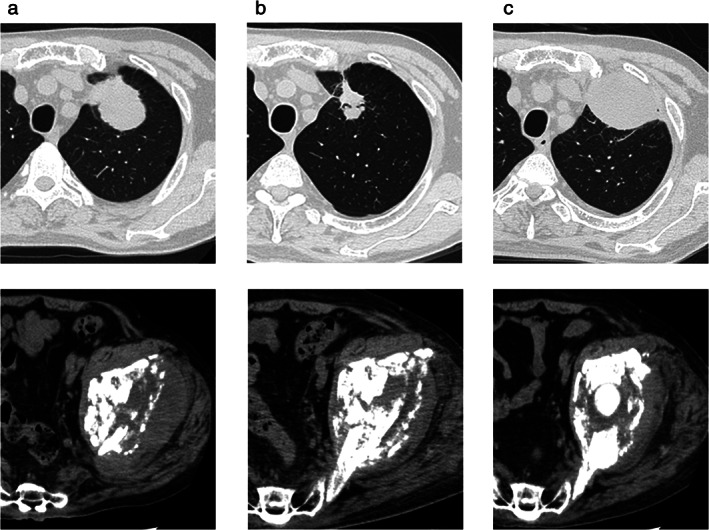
Chest and pelvis computed tomography (CT) scans in a patient with pulmonary sarcomatoid carcinoma. (a) Before treatment with pembrolizumab/carboplatin/pemetrexed, the size of the lung tumor was 50 mm in diameter. (b) After four courses of pembrolizumab/carboplatin/pemetrexed, the diameter of the lung tumor decreased to 26.4 mm. (c) A total of 84 days after initiation of treatment with prednisolone, the diameter of the lung tumor had increased to 62.3 mm. (upper, primary lung tumor; lower, metastasis site of left pelvis, respectively)
